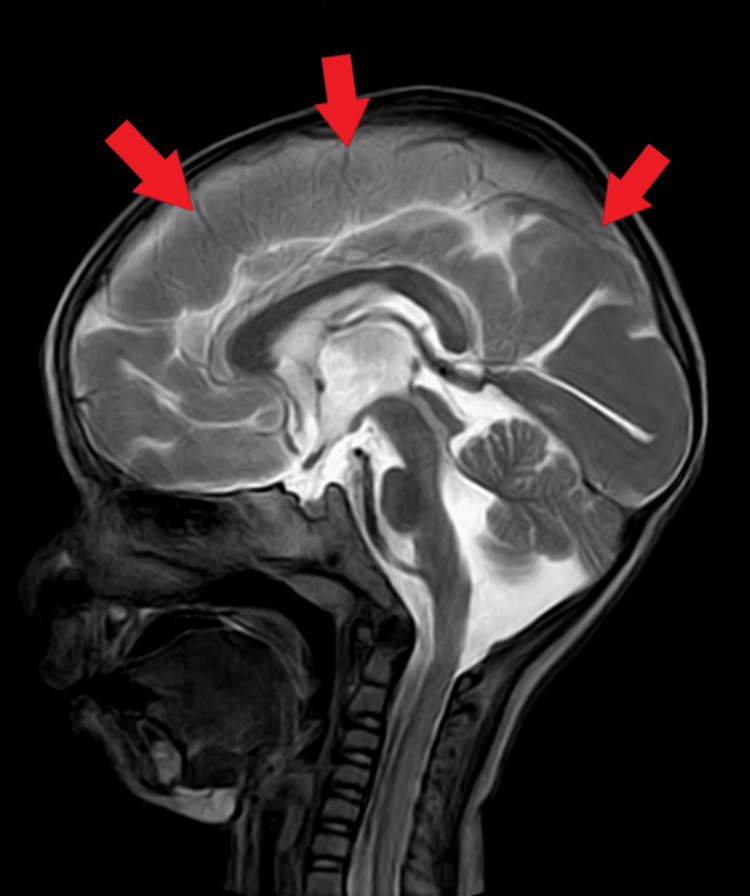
Magnetic resonance image demonstrating mild thickening of the cortex suggestive of type 1 lissencephaly
